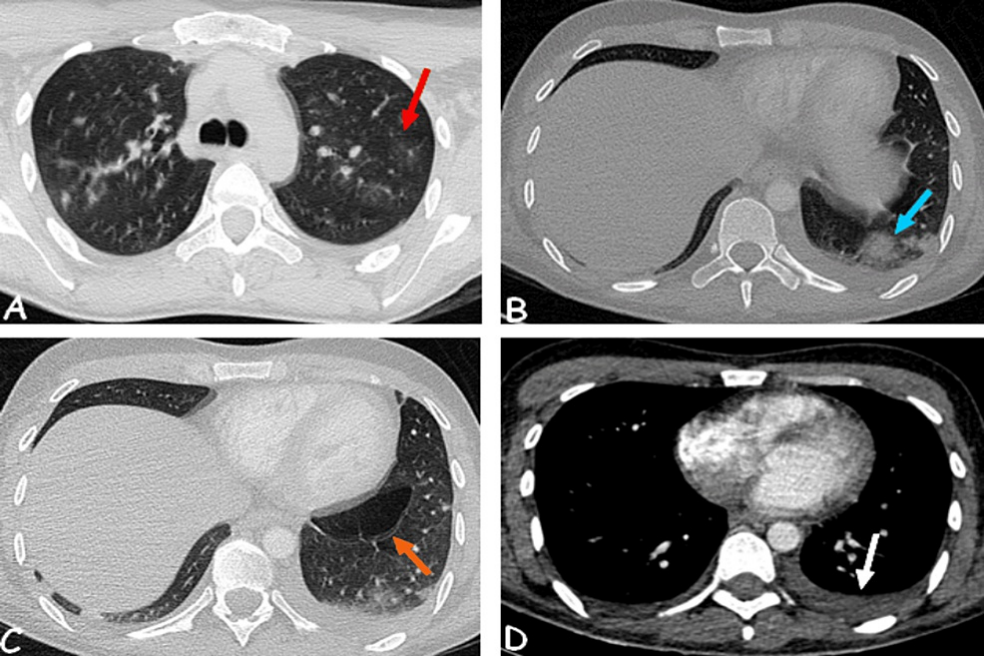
Chest CT scan showing bilateral ground-glass opacities (A), consolidations (B), emphysematous bullae (C), and pleural effusion (D). Red arrow: ground-glass pattern. Blue arrow: pulmonary consolidation. Orange arrow: emphysematous bullae. White arrow: left pleural effusion.
